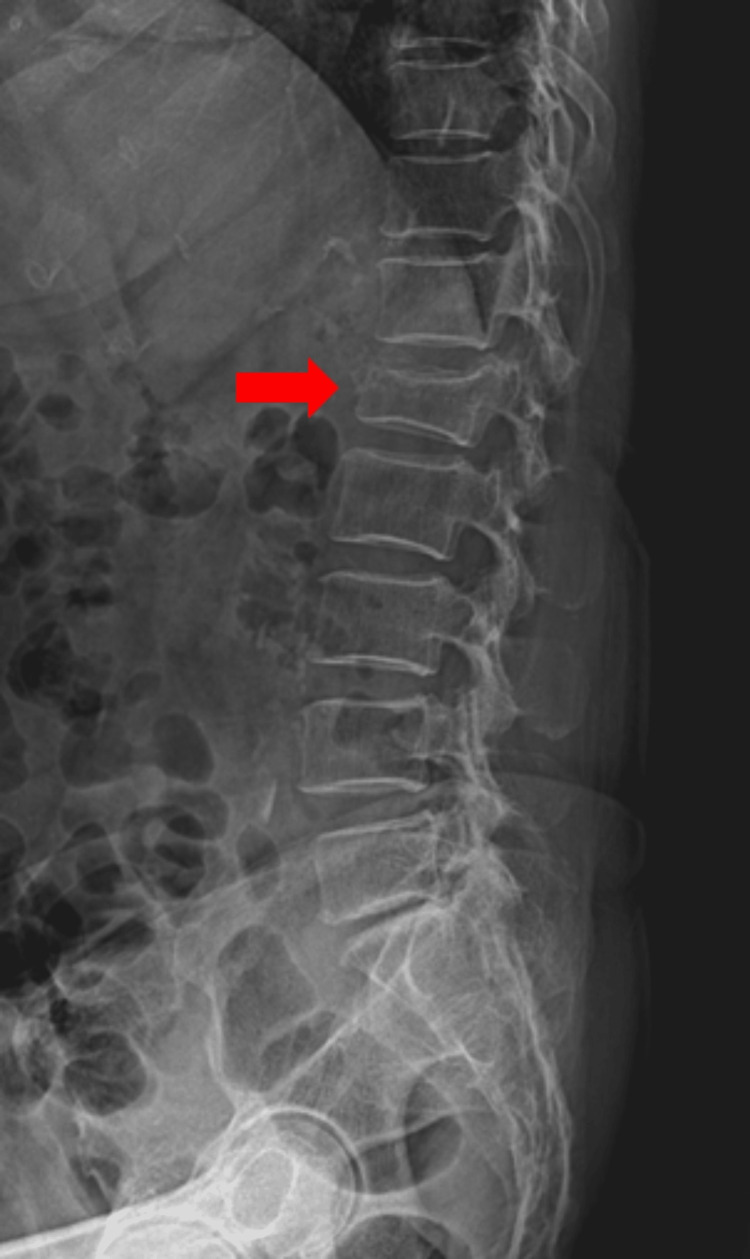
Sagittal plain radiograph of lumbar spine: compression fracture of L1 vertebral body (red arrow).
